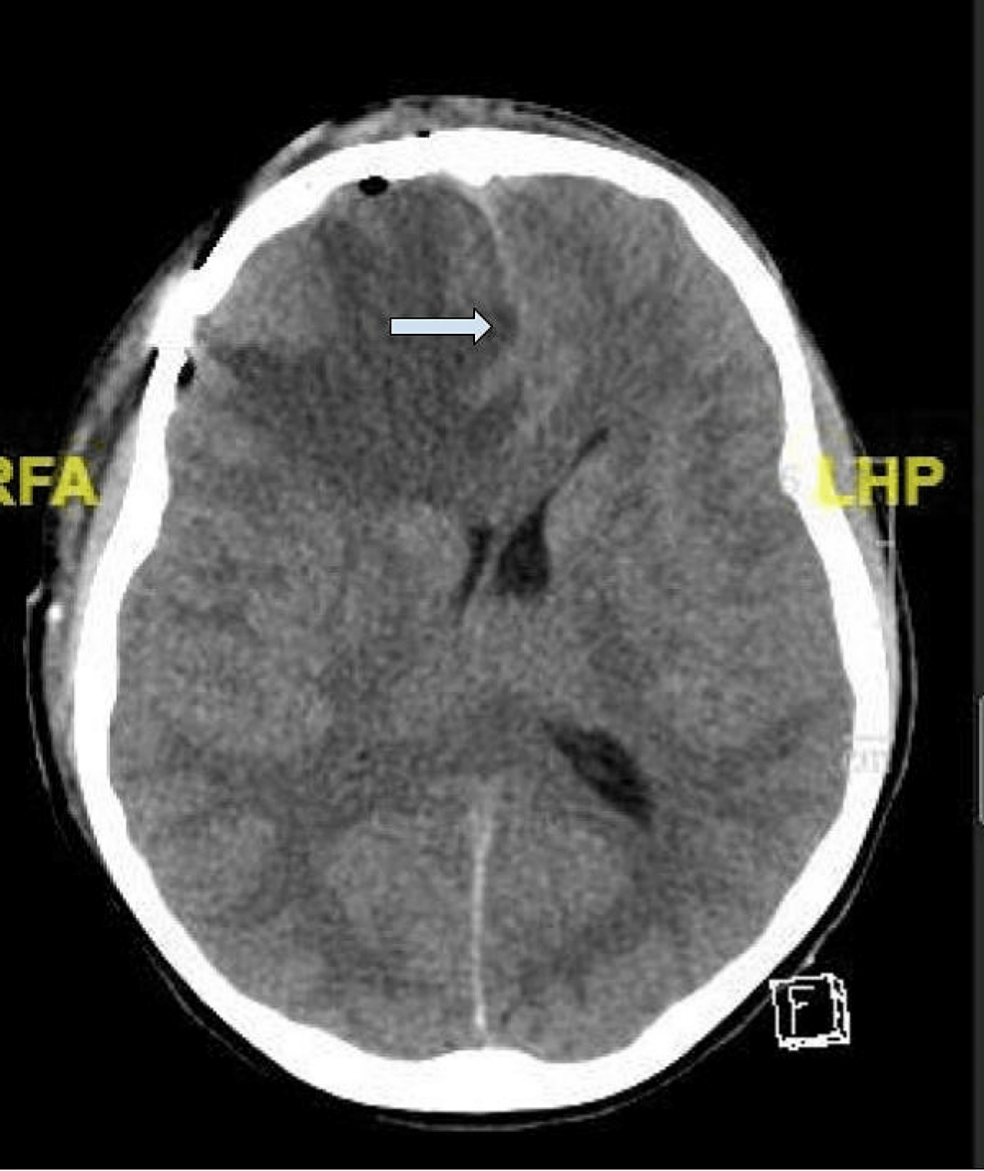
Postoperative day one computed tomography of the brain without contrast showed a mild increase in right anterior frontal lobe vasogenic edema and subfalcine herniation with a right to left midline shift before decompressive craniectomy (symptom day 23).
